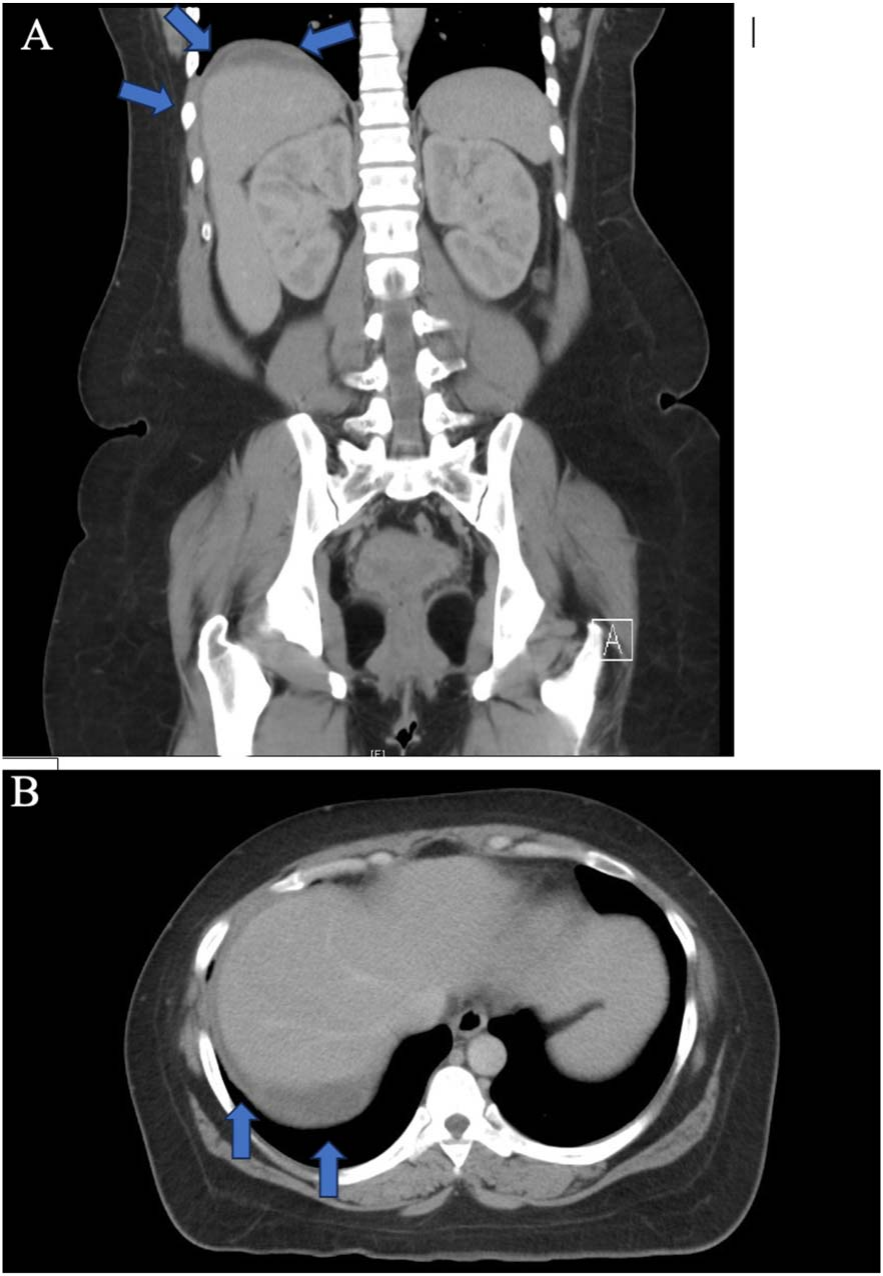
Frontal (A) and transverse (B) planes of computed tomography imaging of the liver of a patient with subcapsular hematoma (*arrows*).
